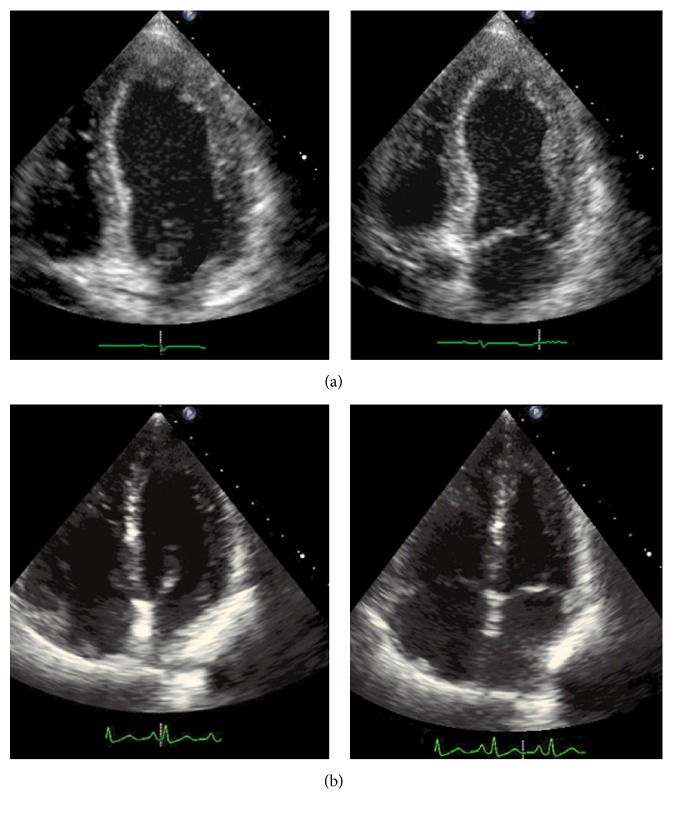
Echocardiogram images obtained during the hospitalization (a) and ten weeks later (b). An apical four-chamber view is shown at end diastole (left) and at end systole (right).
